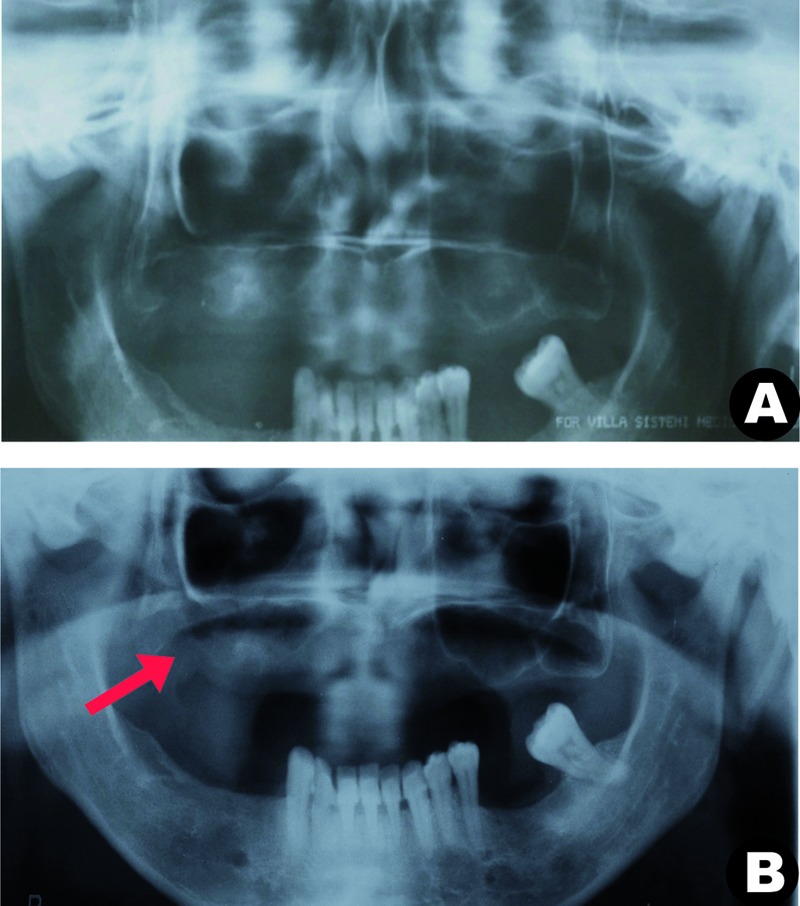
(a) Panoramic radiograph 15 days after surgery; (b, red arrow) showed a partial opacity of the maxillary sinus, compatible with inflammation in the region in remission, and follow-up after 4 months, where a thin radiopaque line of the lower cortical layer of the maxillary sinus can be observed.
